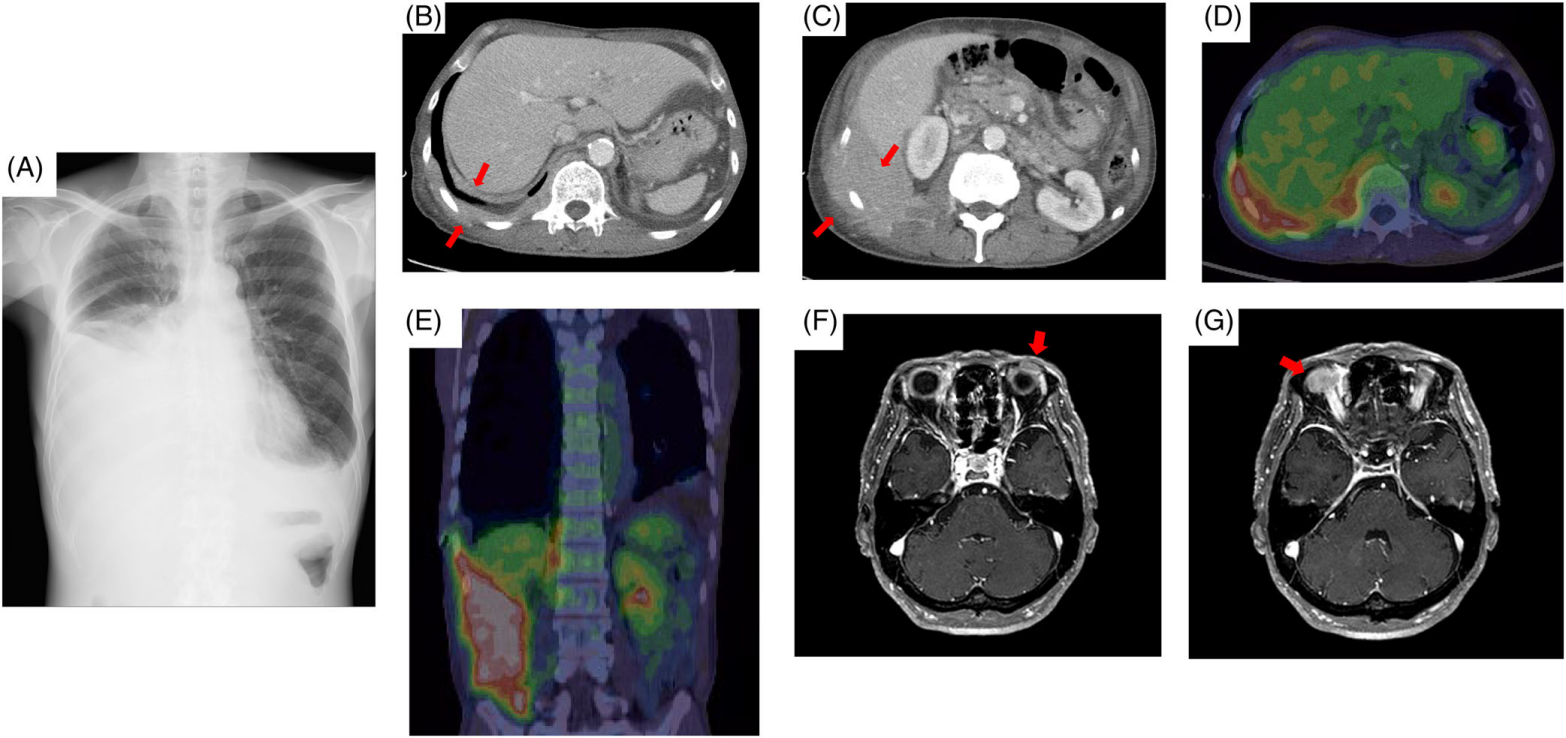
Diagnostic imaging of the patient (A) Chest radiograph shows moderate pleural effusion of the right thoracic cavity. (B, C) Chest computed tomography (CT) scan revealed a right pleural thickening and mass in the retroperitoneum, which spread from the muscular layer to the subcutaneous layer. The image was scanned after thoracic drainage was completed. (D, E) Positron emission tomography (PET) imaging demonstrated increased accumulation of 2‐deoxy‐2‐[18F] fluoro‐d‐glucose (^18^F‐FDG) in the right pleural thickening and the mass of the right retroperitoneum with maximal standardized uptake value (SUV_max_) of 10.51. (F, G) Thickening of the bilateral bulbar conjunctiva on magnetic resonance imaging (MRI) (arrow)
